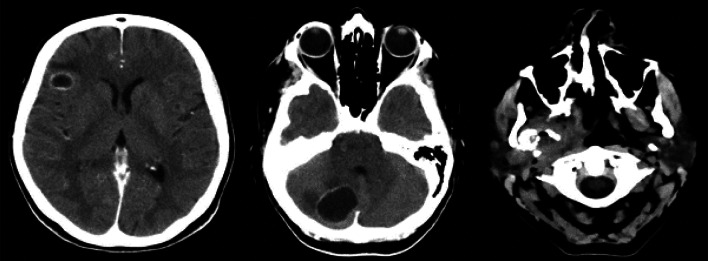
CT scan of the brain and maxillo-facial. The presence of a space-occupying lesion in the right pharyngeal space was confirmed; two lesions were visible at the cerebellar and right frontal level; they presented peripheral enhancement, thus inducing a differential diagnosis between infectious/abscess and neoplastic lesions
